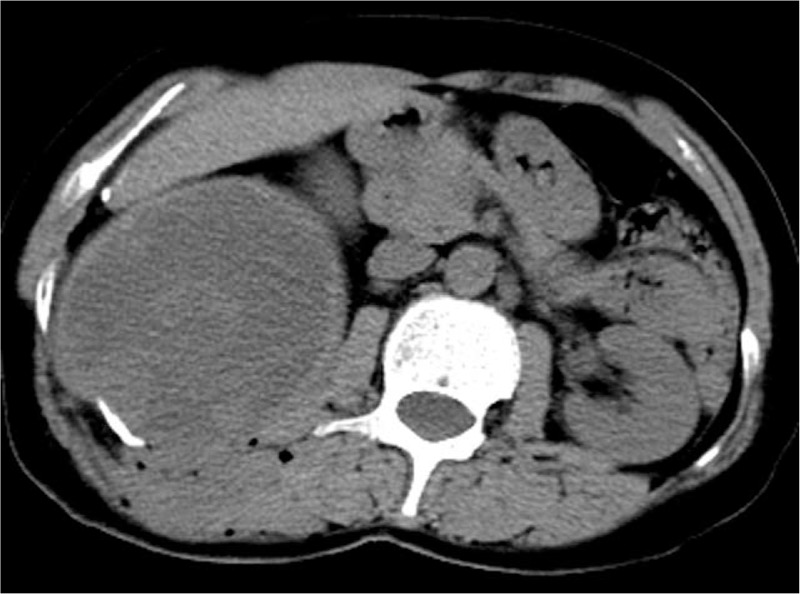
Plain CT scans of the right lower back muscle soft tissue and retroperitoneal low-density cystic mass, the soft tissue nodular wall can be almost seen.
